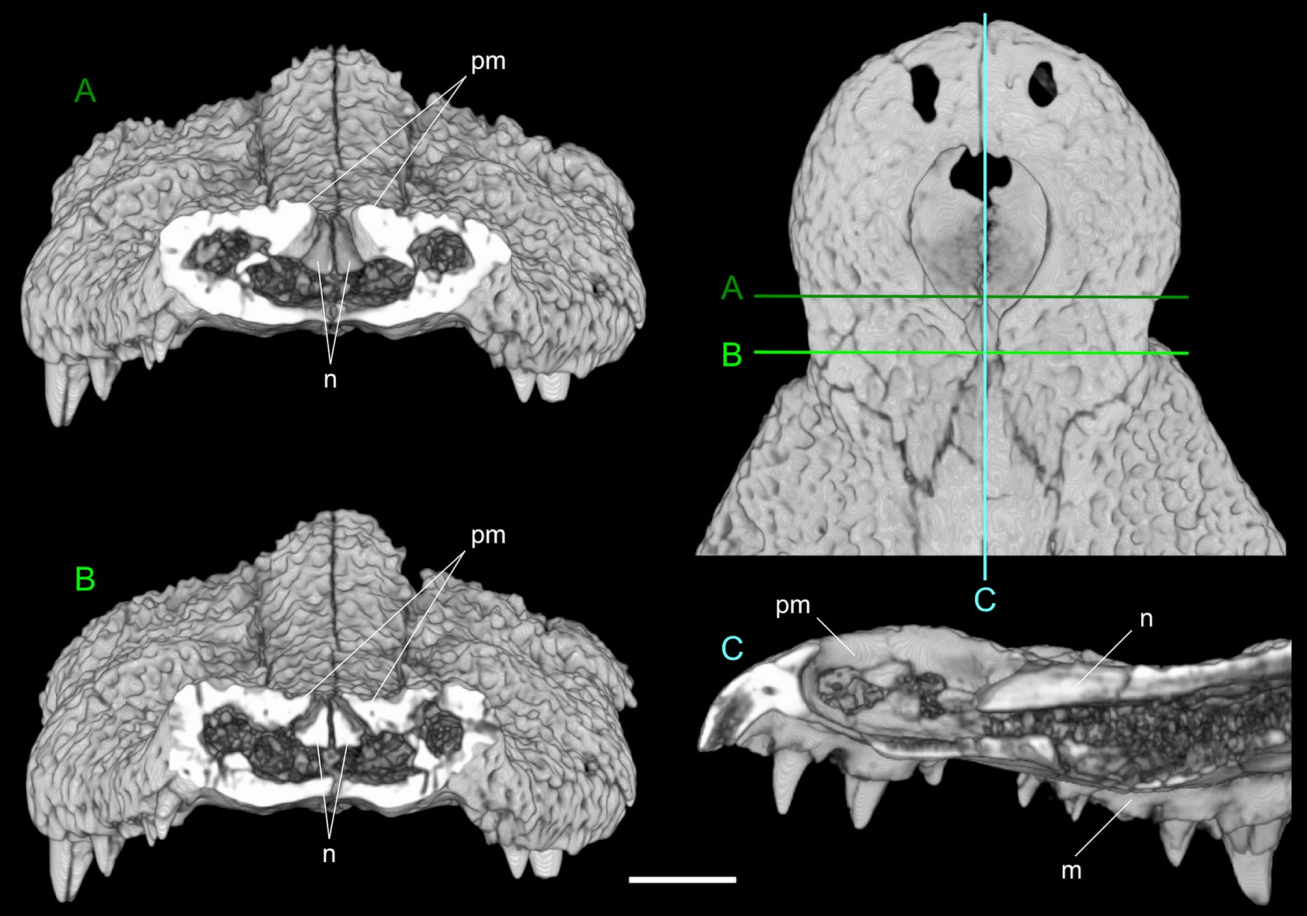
CT investigation of the relationship between the nasals and the premaxillae in sn813/lj. Top left: anterior tip of the snout in dorsal view showing the planes of the sections in (A), (B) and (C). (A) anterior transversal section do not involve the nasals; (B): posterior transversal section cutting the nasals and showing that the premaxillae are entirely separated by the nasals; (C): sagittal section showing that the nasals are slightly lowered in the area of the posterior process of the premaxillae. Note in (A) and (B) how the nasals are raised to form the medial boss of the snout. Scale bar: 3 cm.
